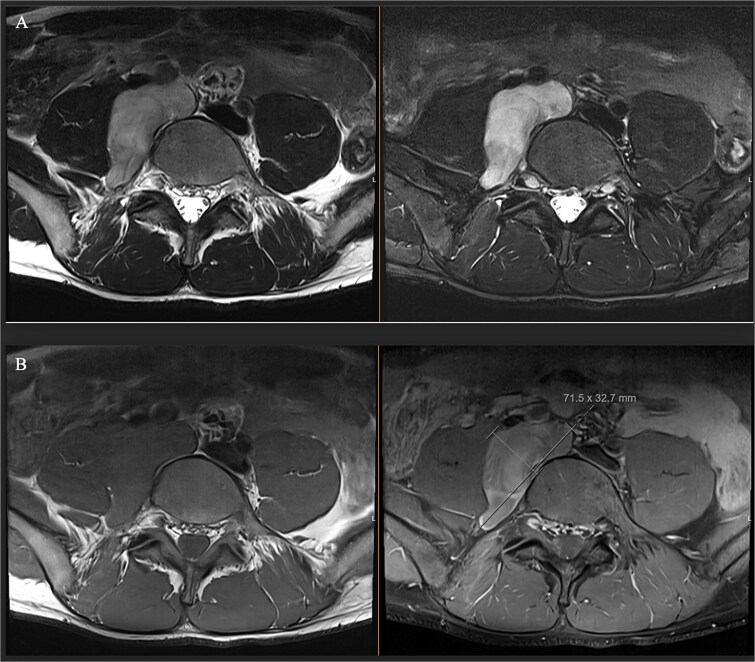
(A) Axial T1 MRI: Left) pre-contrast, right) post contrast demonstrating mild contrast enhancement. (B) Axial T2 MRI: Left) axial T2, right) axial T2 with fat saturation showing hypertense lesion displacing iliac vessels and psoas.
